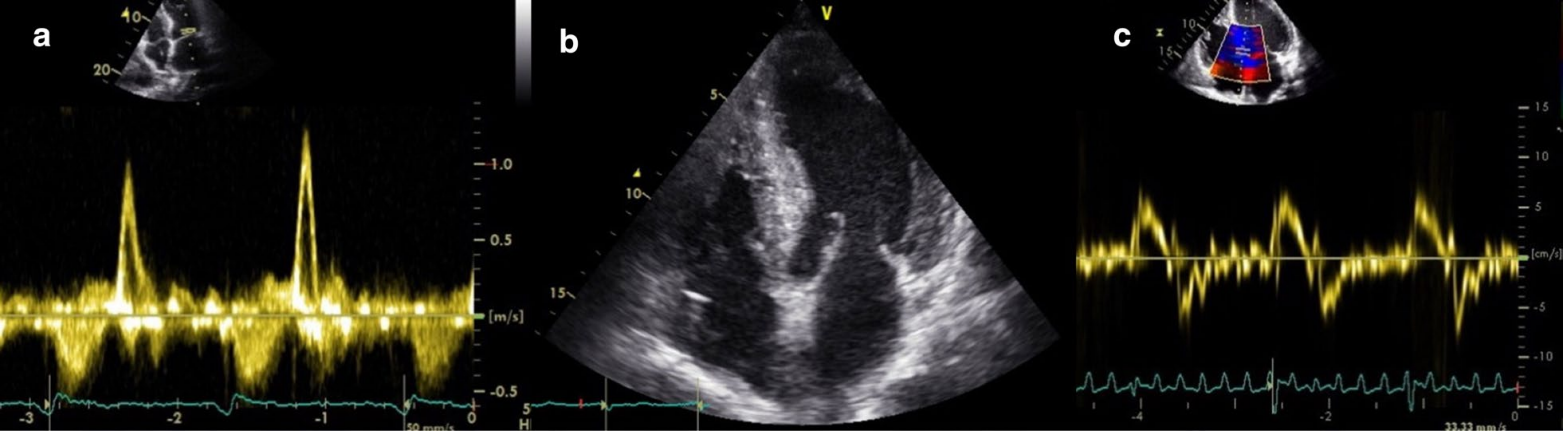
Echocardiographic results. Echocardiographic images showing a restrictive pattern of LV filling, characterized by a high E wave and reduced deceleration time on diastolic mitral valve inflow (a); biventricular hypertrophy and biatrial enlargement are appreciable in b; signs of high filling pressures, demonstrated by a reduced e′ velocity with high E/e′ ratio, are visible on c
